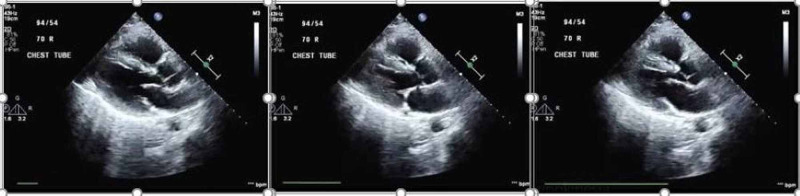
Echocardiogram showing right ventricular free wall hypokinesia
